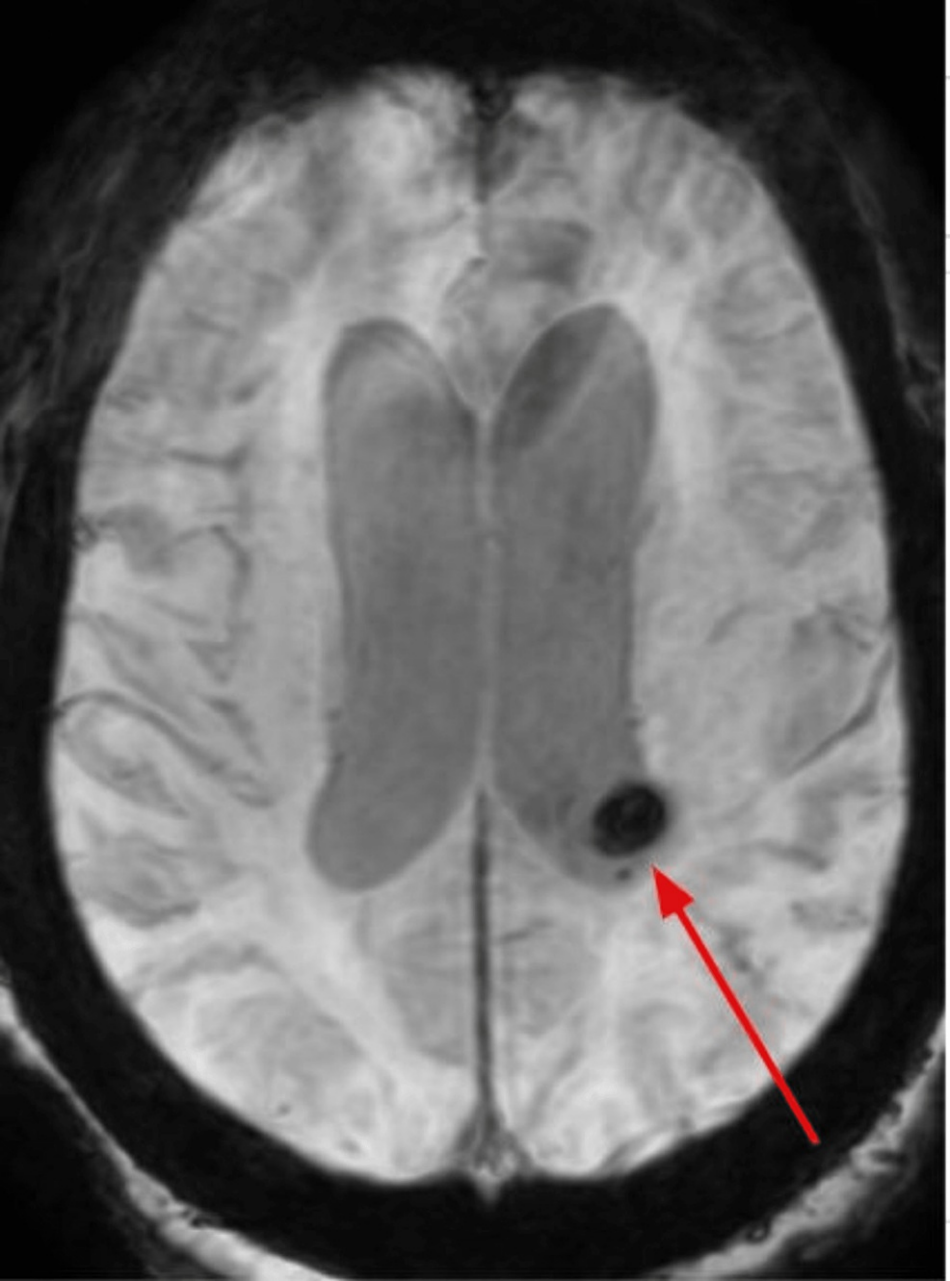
MRI Brain FLAIR showing white matter adjacent to the left lateral ventricle (0.5 cm). These findings are most compatible with a focus of subacute hemorrhage. FLAIR: Fluid-attenuated inversion recovery
